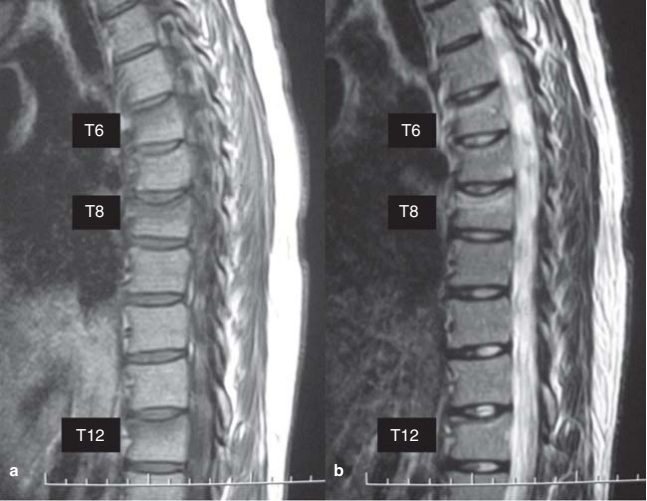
Magnetic resonance imaging (MRI) of the vertebrae in case 4. MRI showed low signal intensities on T1-weighted images (A) and high intensities on T2-weighted images (B) at the 6th, 8th, and 12th thoracic vertebrae.
